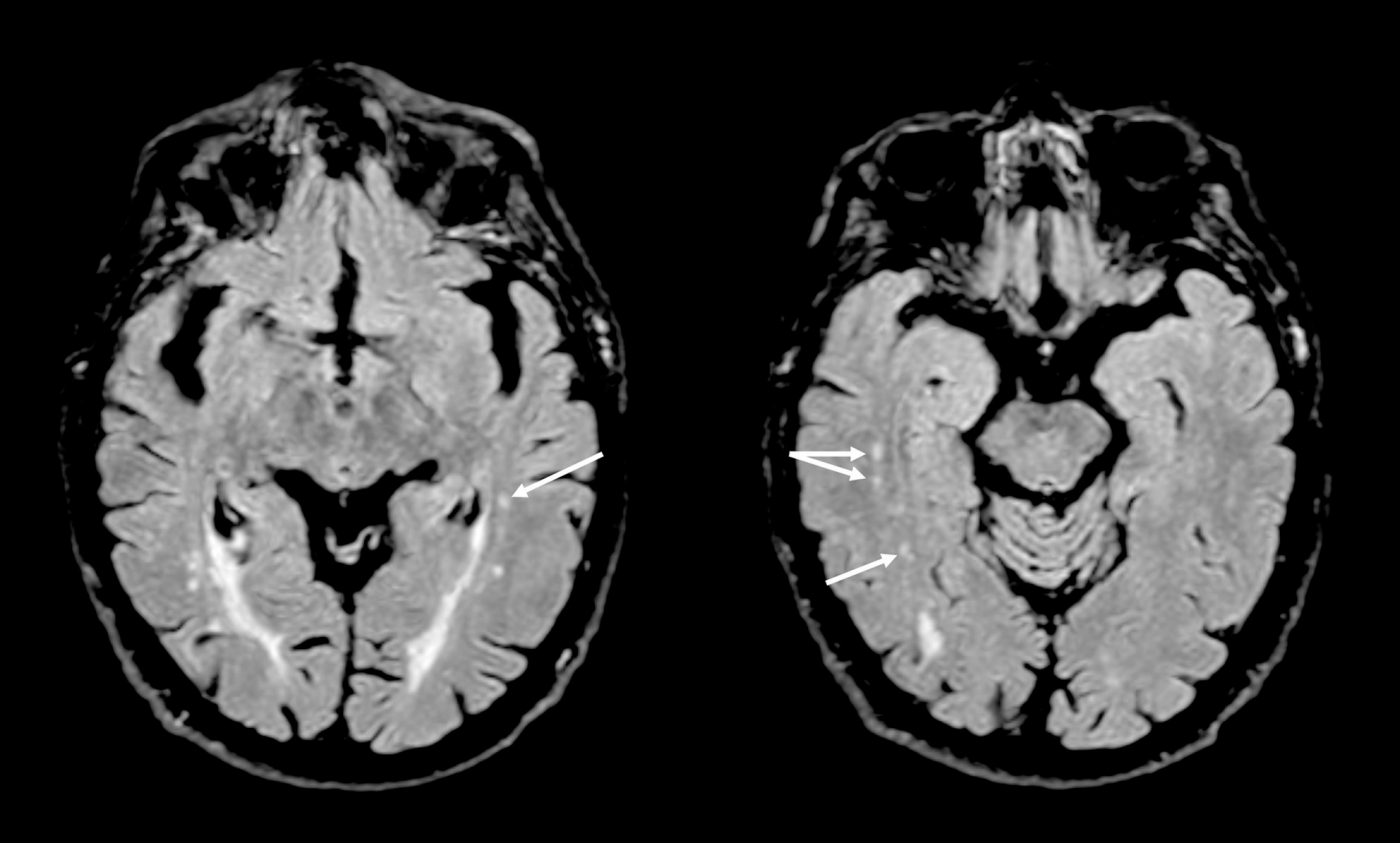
Axial T2-weighted fluid-attenuated inversion recovery (FLAIR) images showing small gliotic foci in deep white matter of both temporal lobes (arrows) in a patient with burning mouth syndrome.
